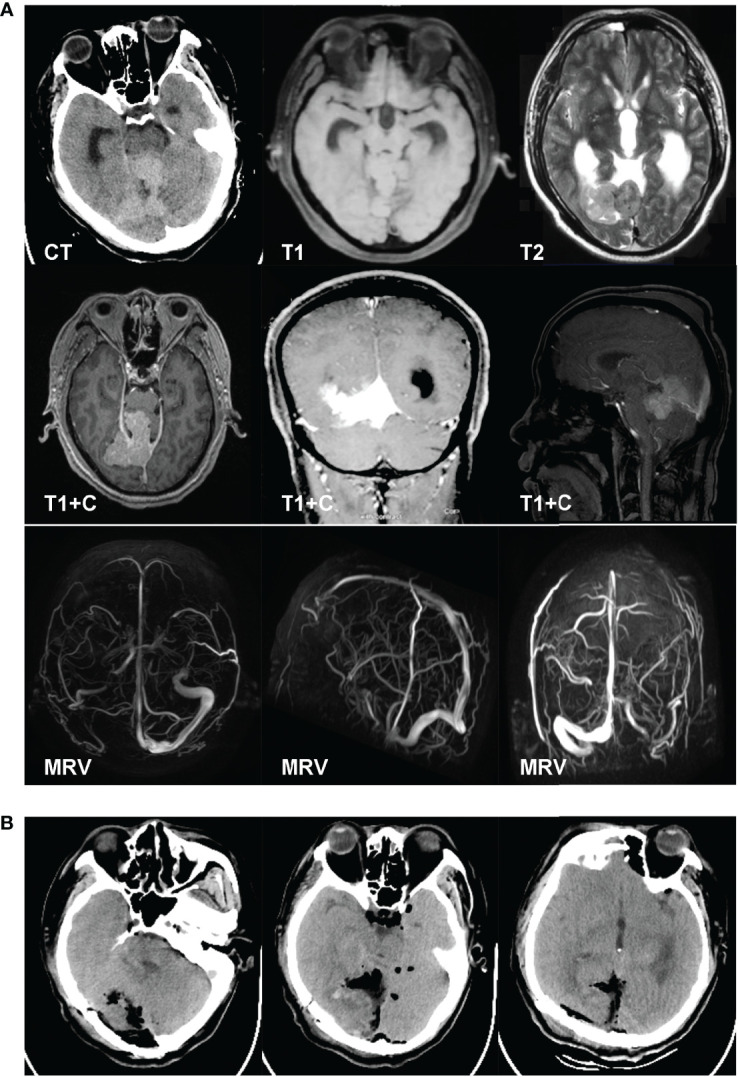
A 52-year-old man complained of blurred vision and unsteady gait for 2 months. The physical examination results showed left hemianopsia, and both VOS and VOD were 0.1. The MRI results revealed a falcotentorial meningioma, which had an isointense signal on T1-/T2-weighted imaging and homogeneous enhancement on contrast-enhanced MRI. The magnetic resonance venography results showed that the straight sinus was occluded (A). We implanted an Ommaya reservoir at 1 week before tumor resection and maintained the drainage at 200 ml per day. The patient then underwent tumor resection using the occipital interhemispheric approach. During the operation, the majority of the tumor base was located at the falx end and the tentorium. The tumor was removed in a piecemeal fashion. Finally, the tumor and the base were totally removed (Simpson grade I resection). The postoperative CT showed no hemorrhage or infarction (B). The pathology examination results revealed that the tumor was a hemangiopericytoma, WHO grade I.
